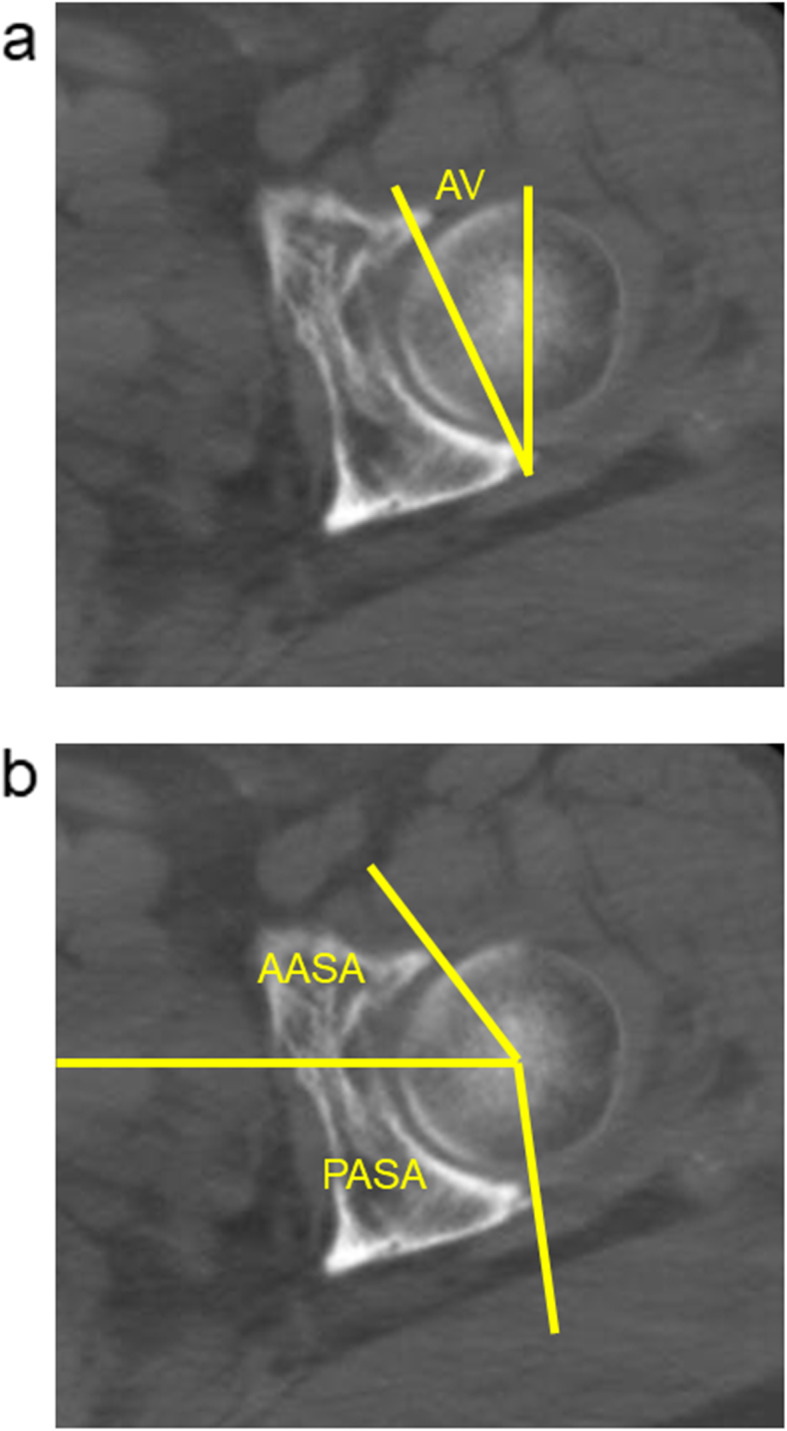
Axial slice of the CT data at the level of the femoral head center. a Measurement of the acetabular anteversion (AV). b Measurement of anterior acetabular sector angle (AASA) and posterior acetabular sector angle (PASA)
